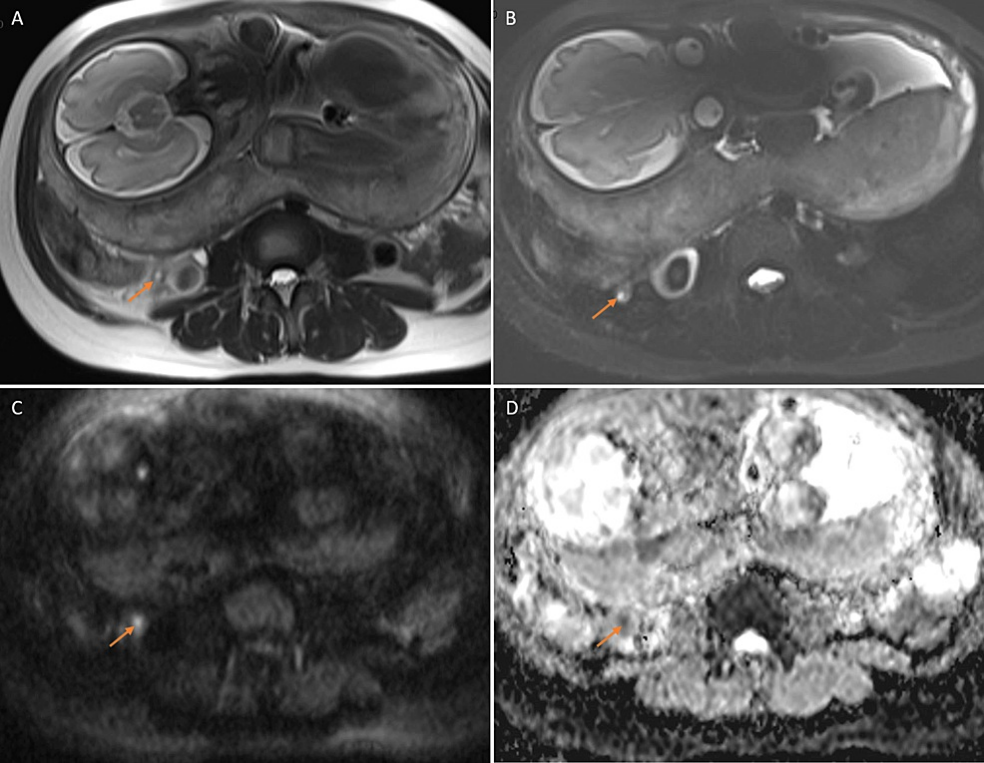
Appendicitis on T2W and DW sequences T2W (A), T2W HASTE TIRM (B), high b-value (800 s/mm2) DW (C), and ADC map (D) images showing the supporting value of diffusion images in characterizing the appendix in a case of surgically and pathologically confirmed appendicitis with undetermined features on T2W images. The appendix (arrow) had a diameter of 8 mm and very mild surrounding stranding. Appendiceal restricted diffusion was evident: hyperintense on DW images and correspondingly hypointense on the ADC map. T2W: T2-weighted, HASTE TIRM: half-Fourier single-shot turbo spin-echo short tau inversion recovery, DW: diffusion-weighted, ADC: apparent diffusion coefficient
